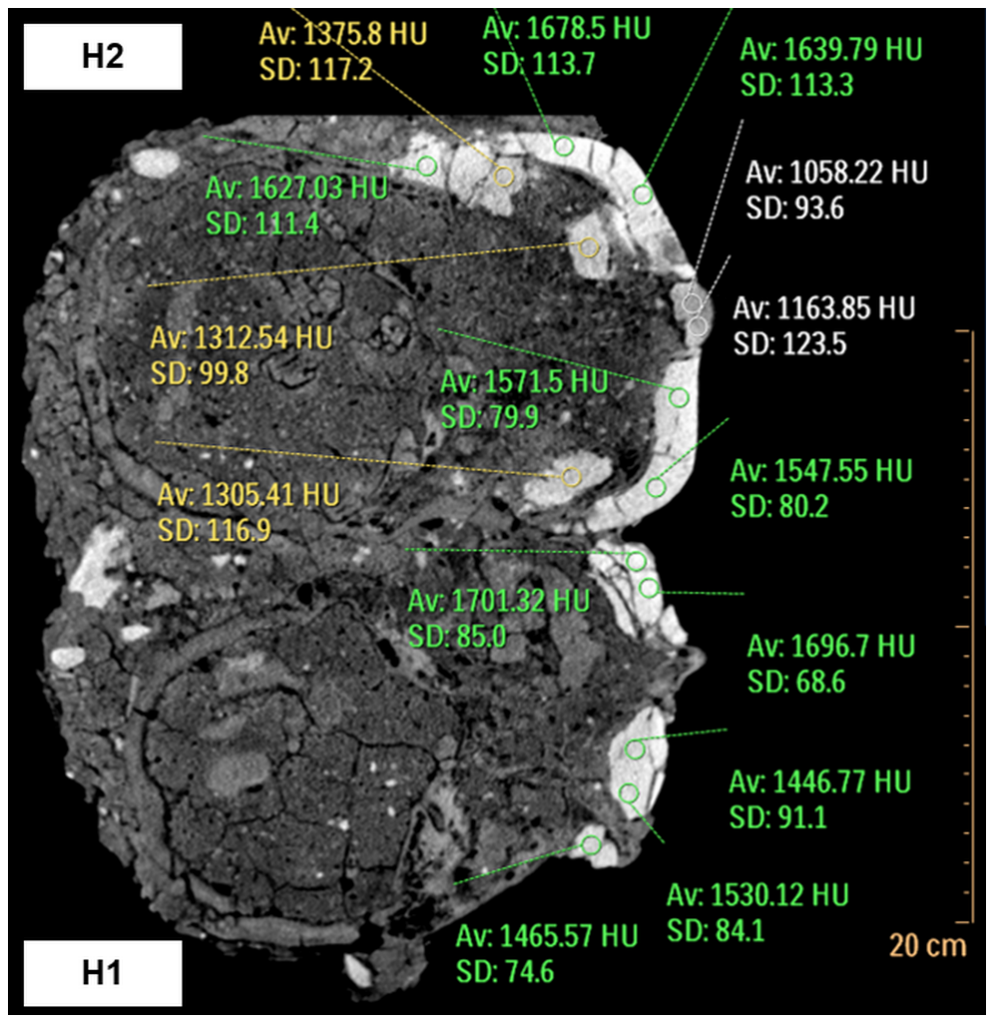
CT scan of Homo 1 and Homo 2. Scans of Homo 1 (lower half of figure) and Homo 2 (upper half of figure) showing results of the ROI analysis, indicating the density of the plastered masks. Densities below 1200HU are marked in white; between 1200–1400HU in yellow; and above 1400HU in green.
